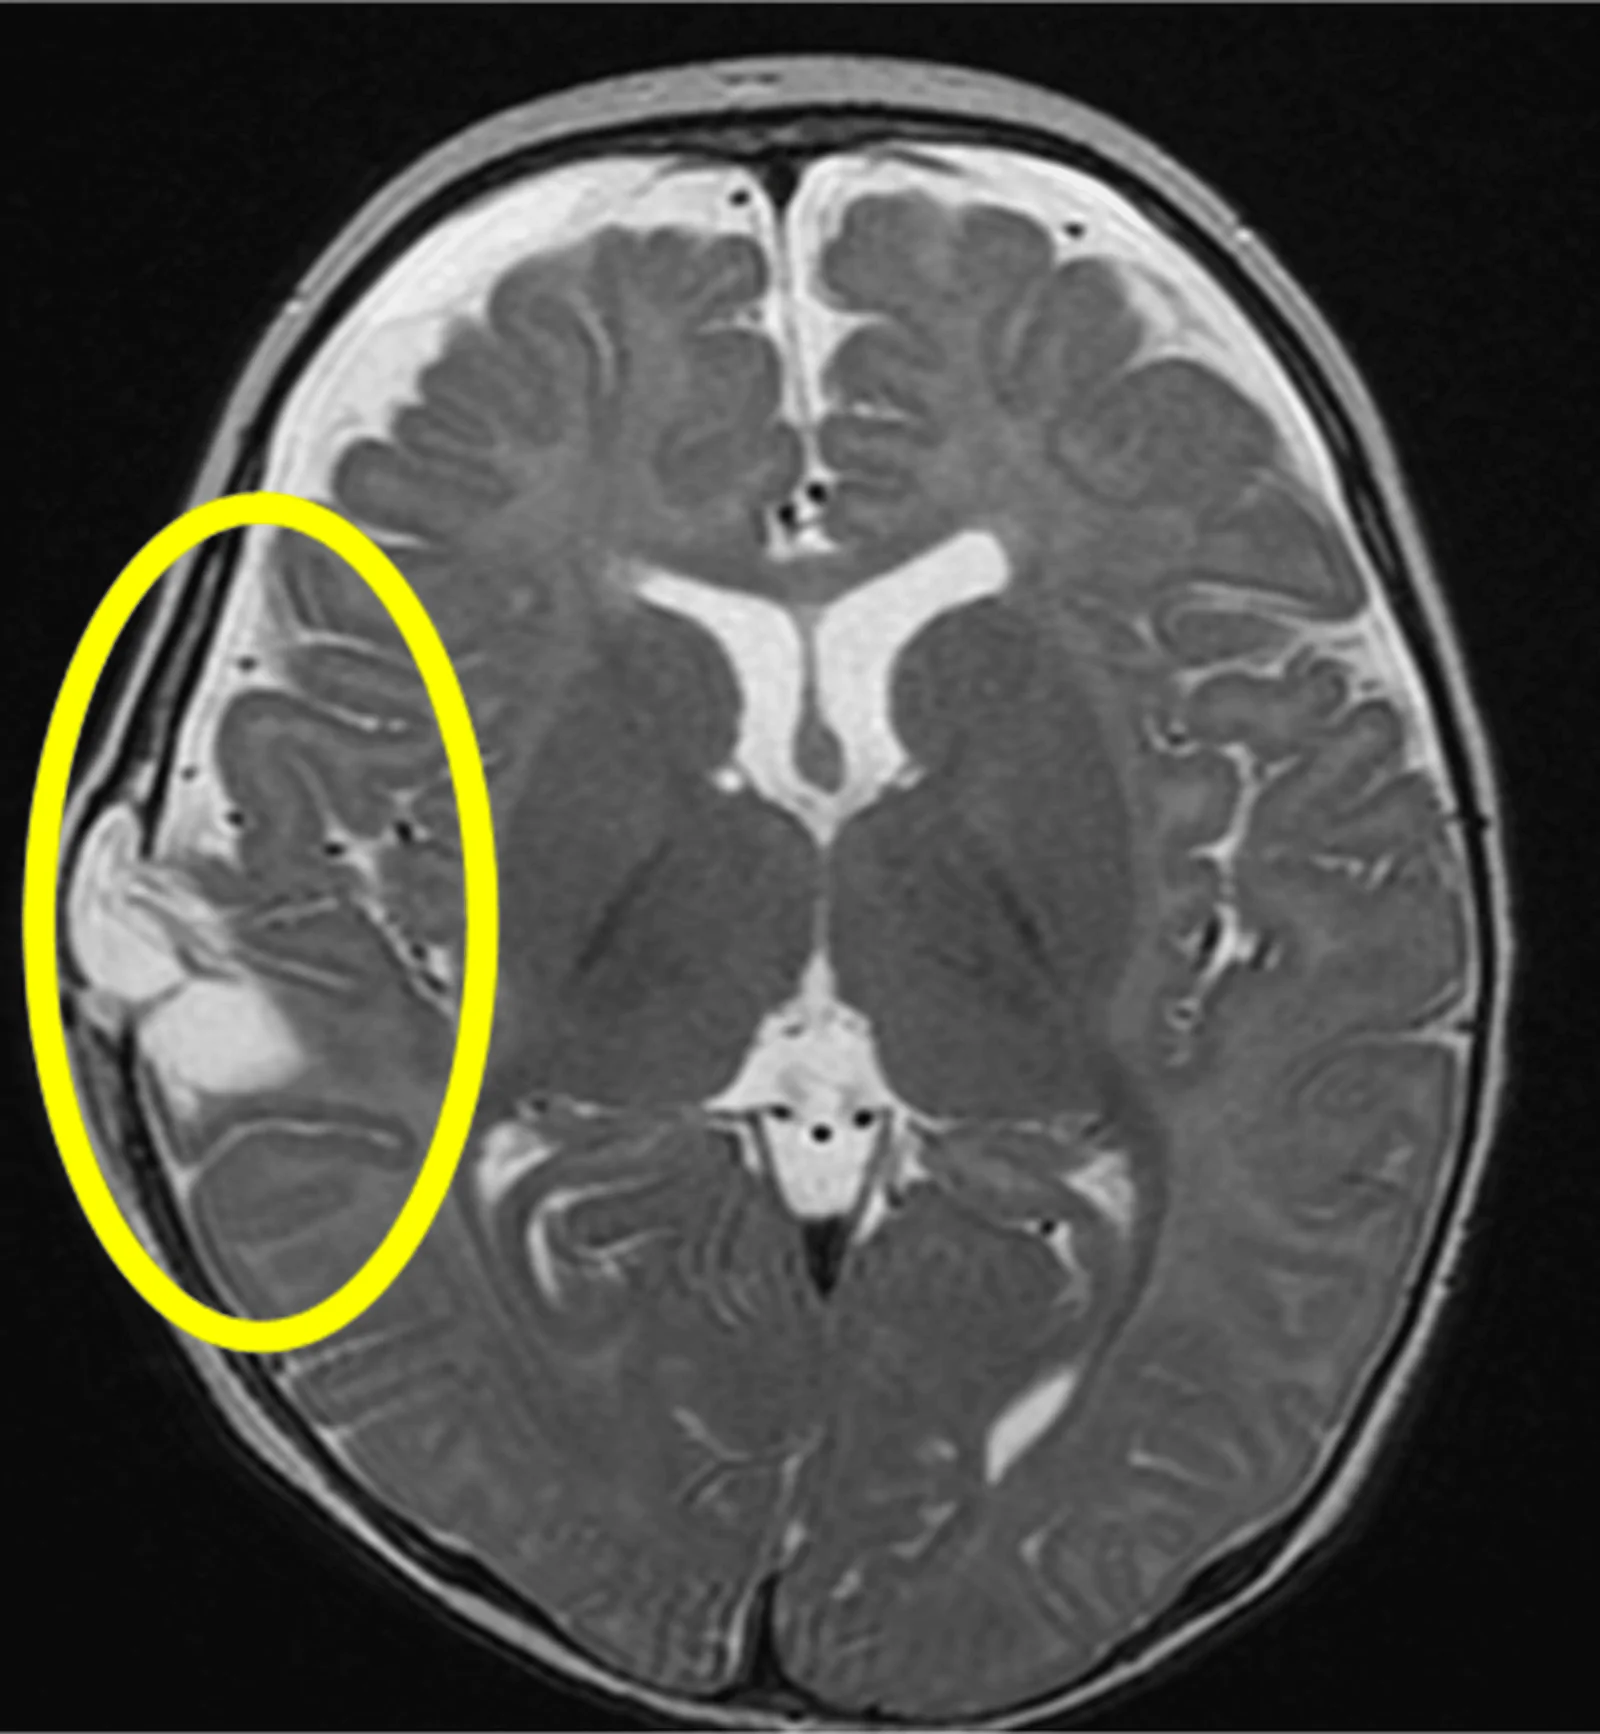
Axial T2-weighted magnetic resonance imaging obtained under sedation on day 48 post-injury Clear dural discontinuity accompanied by a newly developed cystic lesion and brain herniation at the fracture site (circle), supporting the definitive diagnosis of a growing skull fracture.
